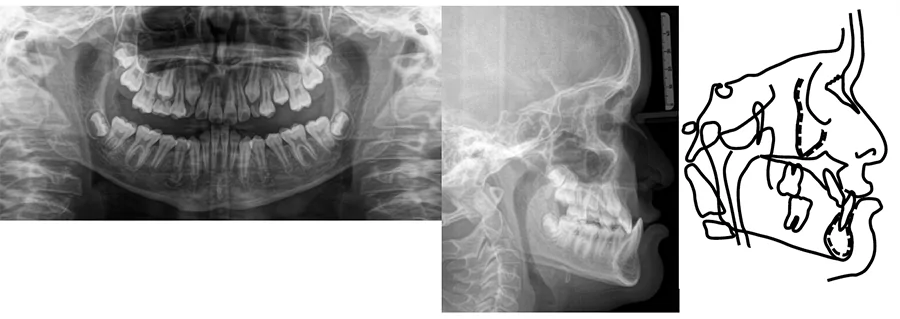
Pretreatment panoramic and lateral cephalometric radiographs, and cephalometric tracing.
